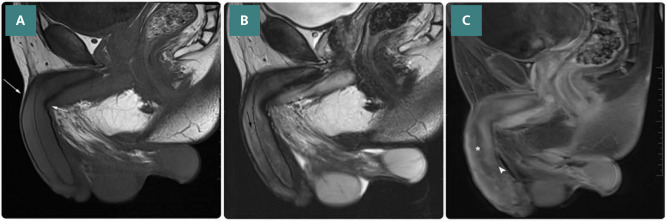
Sagittal MRI scan of the penis. A. T1-weighted spin-echo sequence shows counterclockwisetunical narrowing and torsional twisting at the dorsal and ventral aspects of the proximal penile shaft (white arrows). No fascial discontinuity or uplifting of the superior dorsal vein are noted. B. T2-weighted spin-echo sequence shows heterogenous, multifocal, high-signal intensities distributed across all fascial layers at the dorsal aspect of the corpora (black arrow). C. T1-weighted post-contrast image with fat saturation sequence shows heterogeneous enhancement of the corpora and glans penis, corresponding to areas of chronic tissue ischemia (arrowhead). Additionally, the dorsal-covering fascial layers show intense enhancement secondary to contrast engorgement within the superior dorsal vein. A corresponding lack of central enhancement of the spongy urethra (asterisk) is noted, indicating necrosis as a sequela of chronic ischemia.
